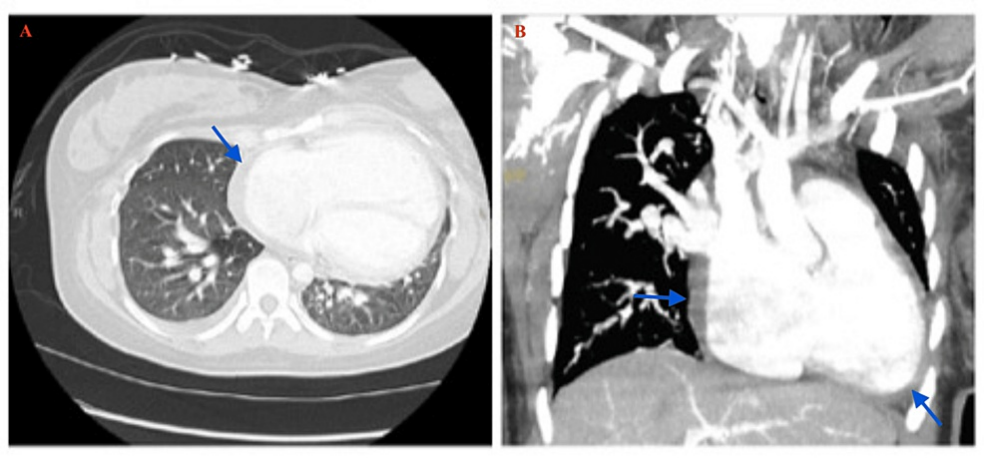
CT angiography (CTA) Chest This is a CTA chest demonstrates a trace pericardial effusion along with atrial septal defect (ASD).  Figure A is the CTA on the axial plane. Figure B is the coronal view of the CTA.  The blue arrows indicate the trace pericardial effusion. The image was done after pericardiocentesis, in preparation for ASD repair and tricuspid valve annuloplasty.
